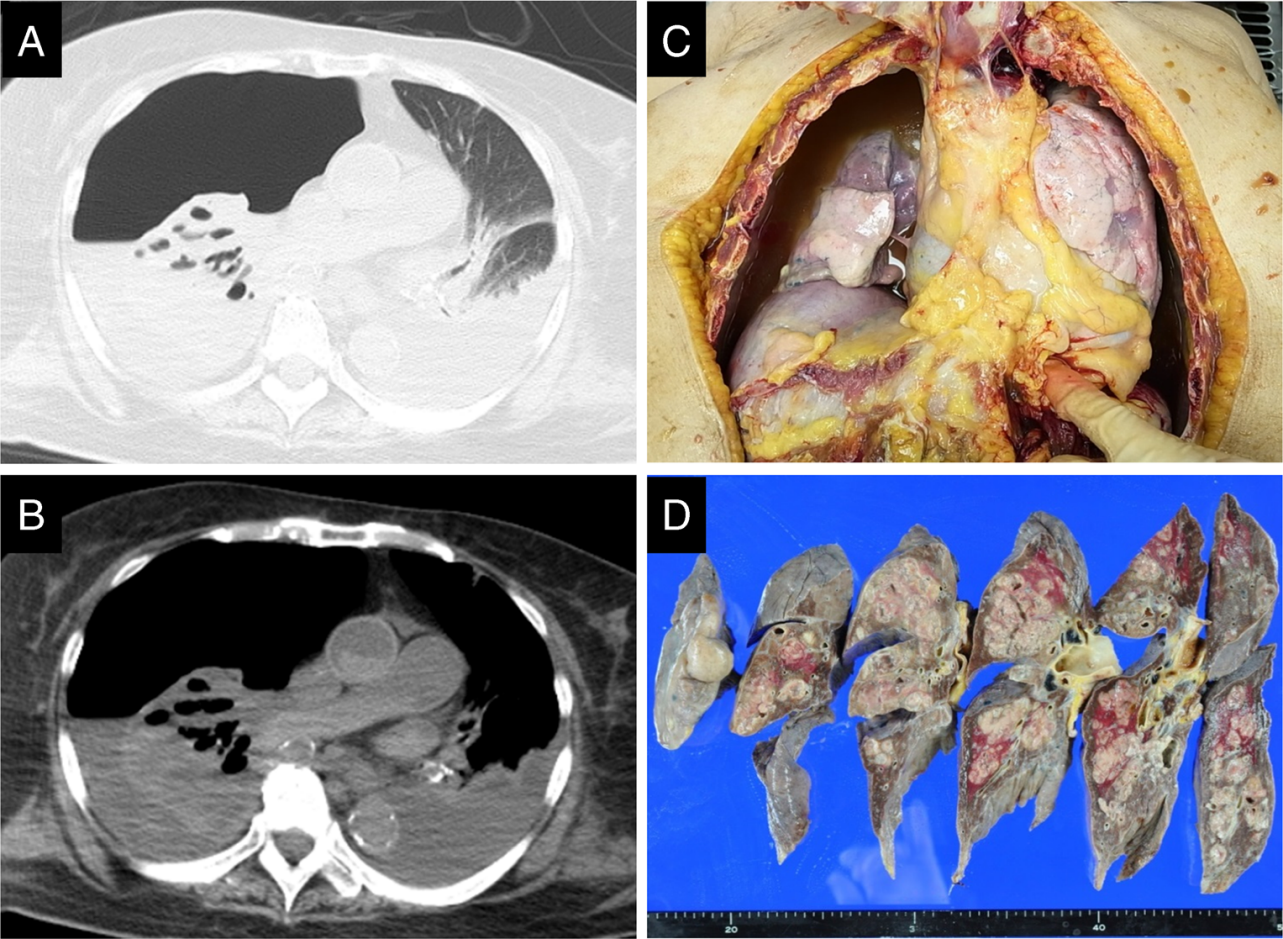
Vanishing pneumothorax by hospital autopsy. Pulmonary window setting (a) and mediastinal window setting (b) demonstrate a pneumothorax in the right lung that was easily detectable by postmortem computed tomography, while the lesion had disappeared during open-chest autopsy (c). The pneumothorax was induced by multiple lung metastases that were only diagnosed by hospital autopsy (d)
